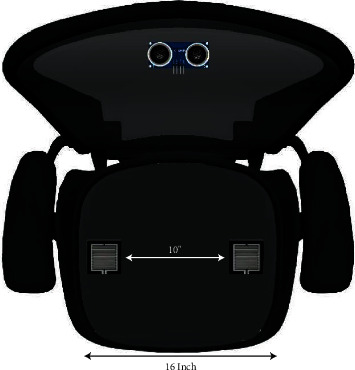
Arrangement 02: two FSR and one ultrasonic sensor.
